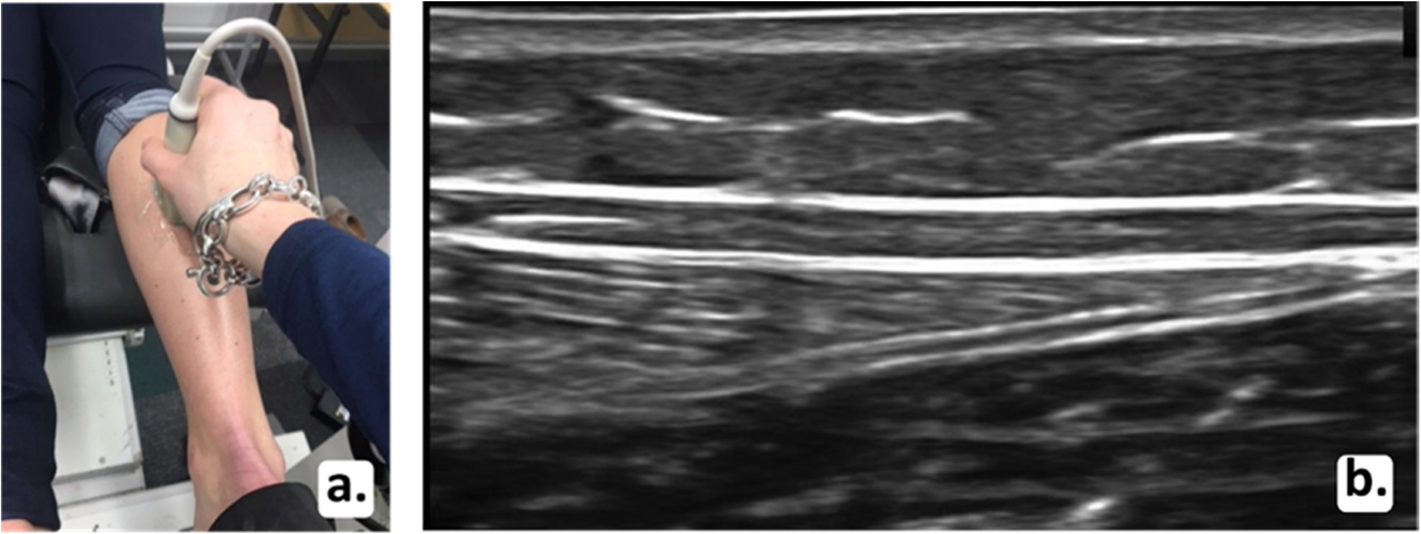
First probe positioning (a) and resulting ultrasound image of musculotendinous junction as indicated by the arrow (b)
